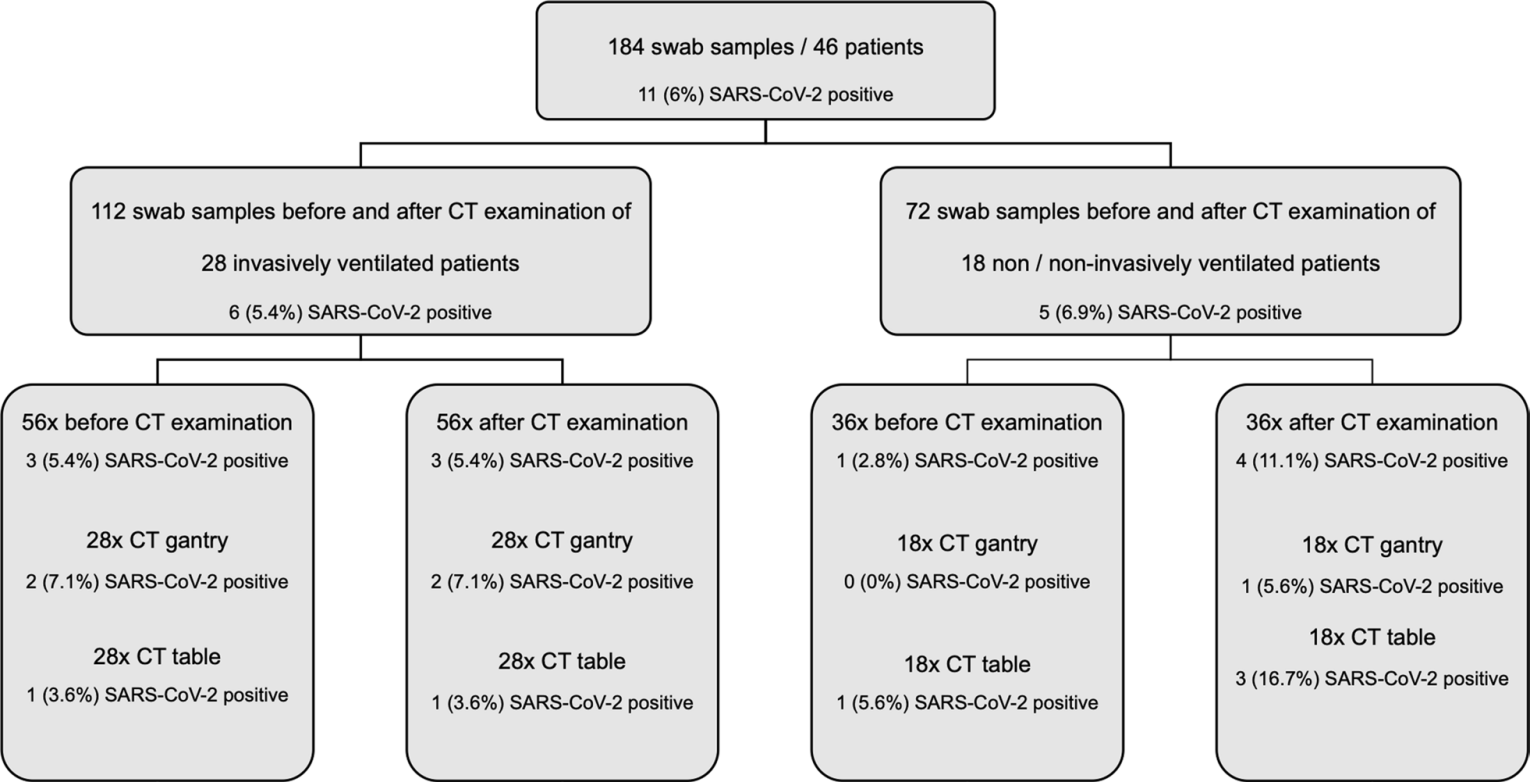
Visualization of the sampling process from patient near surfaces (CT table/CT gantry). SARS-CoV-2: severe acute respiratory syndrome coronavirus type 2; SARS-CoV-2 positive: number and percentage of positive RT-PCR results for SARS-CoV-2 RNA
